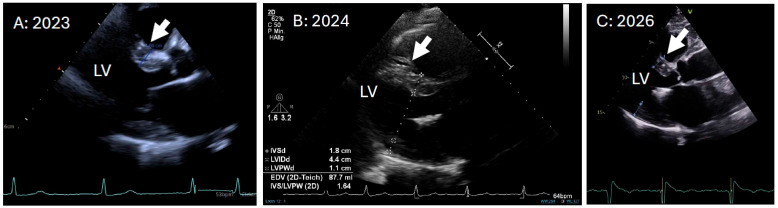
Septal thickening for disease monitoring in echocardiography. Although septal thickness is of limited use, it is recommended for monitoring and can indicate slow changes over time. Attention should be paid to minor measurement-related inaccuracies. (A) 2023 parasternal long axis before diagnosis (19 mm). (B) 2024 parasternal long axis after diagnosis and before start of therapy (18 mm). (C) 2026 parasternal long axis 2 years after start of therapy (15 mm). White arrow: septal thickening. LV: left ventricle.
